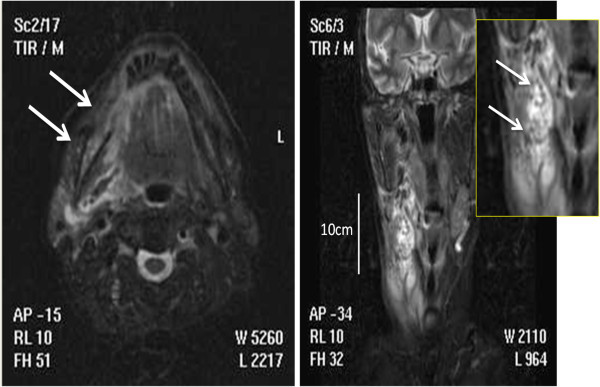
Magnetic resonance imaging (MRI) study of head and neck. A, Axial MRI scan showing a significantly increased signal of the bone marrow lesion (yellow arrow) when compared with left mandibular body (asterisk), with bone edema indicative of an inflammatory process taking place in the right mandibular body and gas tracking along the two pterygoid muscles (white arrows). B, A coronal T2-weighted image of the submandibular region showing a wide mass with very high signal intensity from the parapharyngeal space to the inferior cervical region with associated edema.
